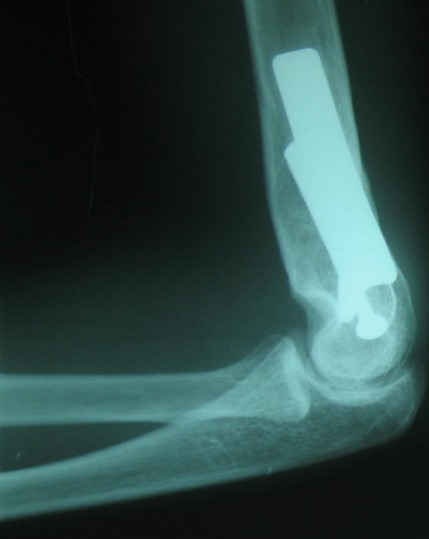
Radiographie de profil du même patient
